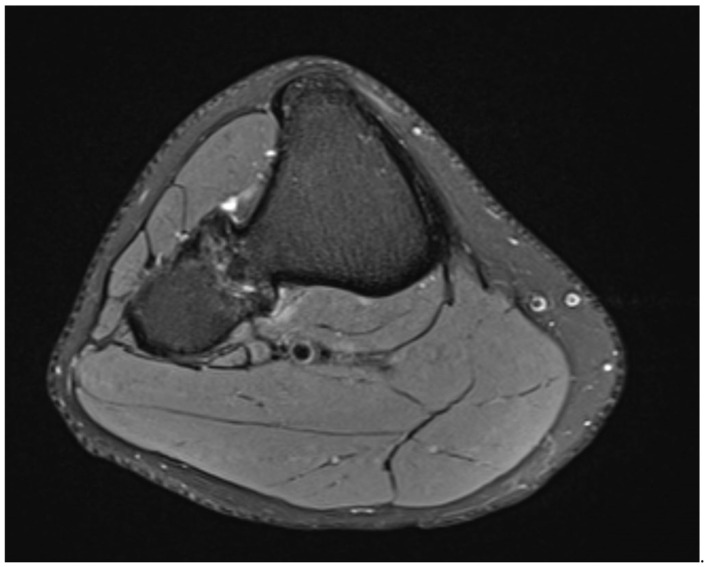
MRI scan in horizontal view showing the synostosis of the right side.
